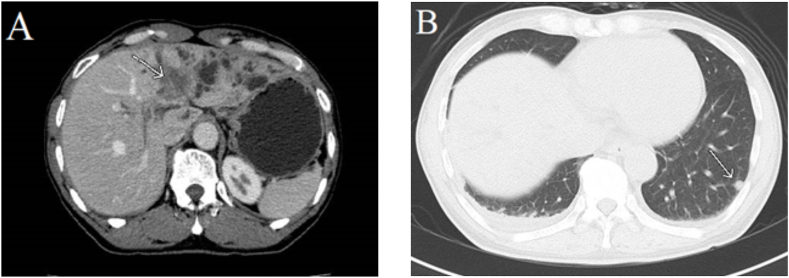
A 59-year-old man with perihilar cholangiocarcinoma. Portovenous phase CT shows an irregular poor enhancing mass at the hilar (white arrow) invading the left portal vein and causing intrahepatic duct dilation and atrophic changes in the left lobe of the liver (Fig. 8A). Post-curative resection follow-up chest CT at 3 months shows evidence of pulmonary metastasis at the lateral segment of the left lower lobe (white arrow; Fig. 8B).
